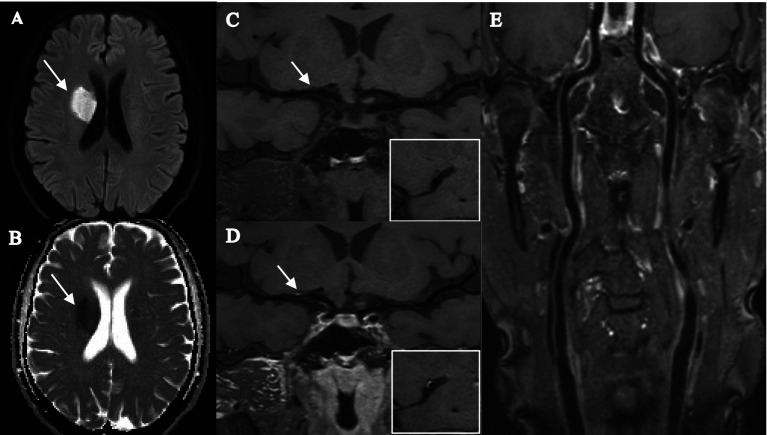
Female, 56 years old, was admitted to the hospital with orofacial tilt and left lower limb weakness for 2 days, and did not experience stroke recurrence during the 12-month follow-up. (A,B) DWI image shows a patchy hyperdense shadow in the right radiocoronal area with a corresponding reduced ADC value (arrow). (C,D) T1-SPACE images before and after enhancement show the presence of plaque in the M1 segment of the right middle cerebral artery (arrow), with low plaque burden, isosignal plaque before enhancement, mild enhancement after enhancement, and mild stenosis. (E) T1-SPACE image of the extracranial carotid artery does not show the presence of plaque.
